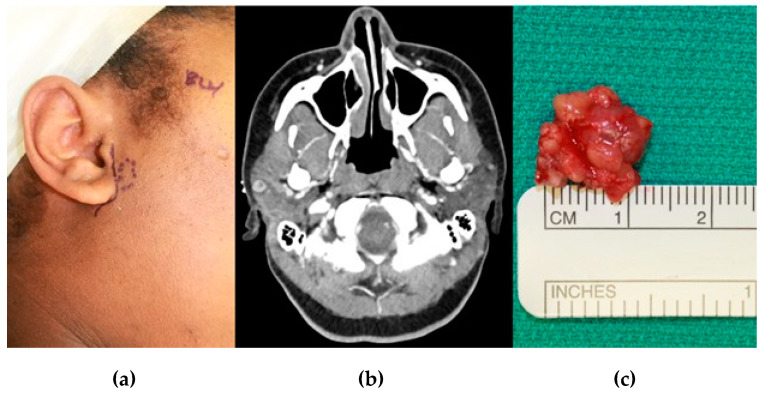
A 26-year-old female presented for evaluation with chief complaints of right TMJ popping and pre-auricular pain for one year. She reported a strong family history of both systemic lupus erythematosus and Sjogren Syndrome. C-reactive protein was elevated and anti-nuclear antibodies showed speckled and homogenous patterns in high titers. (a) Dotted line indicates location of pain per patient; (b) CT was intentionally obtained with contrast to evaluate for TMJ and soft tissue abnormalities. Note an intra-parotid lesion lying immediately lateral to the mandibular condyle; (c) Intra-parotid lesion removed and found to be a basal cell adenoma. The patient’s symptoms resolved after treatment.
